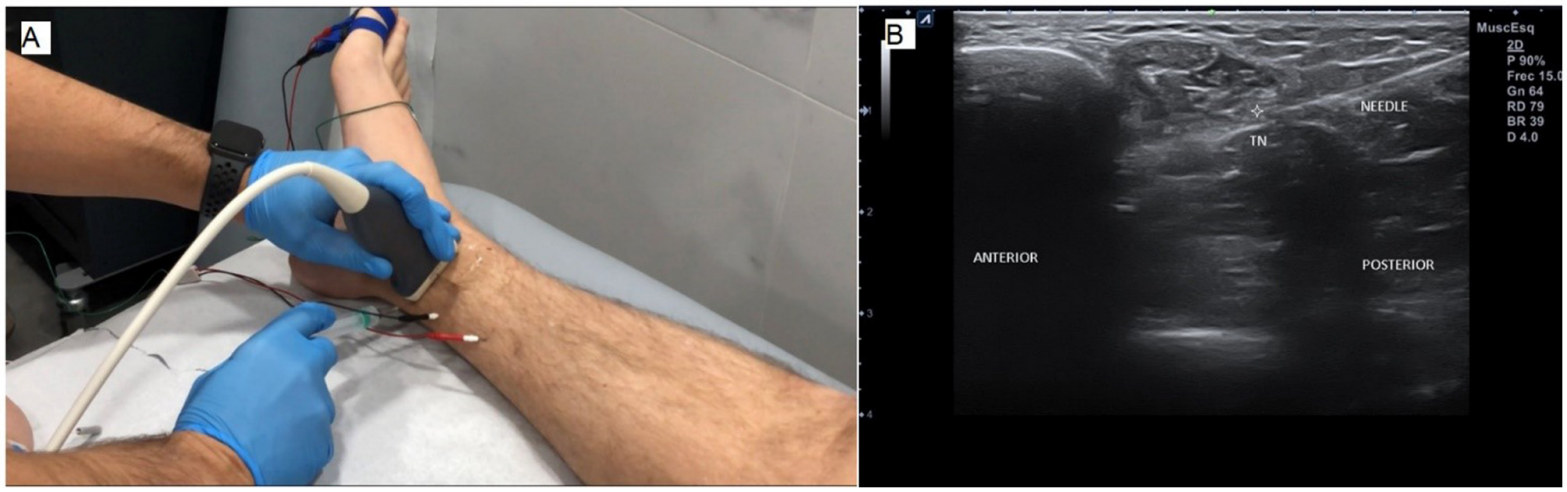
(A) Ultrasound-guided lidocaine infiltration test (USLIT) and the active and reference needle position for recording is displayed. (B) Ultrasound image showing the position of the needle for infiltration with respect to the tibial nerve (TN).
